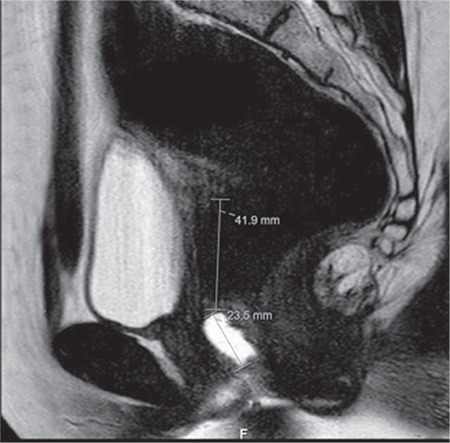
Sagittal view of the magnetic resonance imaging scan after gel injection into the vagina showing the exact level of the septum (A: distance from introitus to septum: 23.5 mm; B: distance from septum to posterior vaginal fornix: 41.9 mm)
